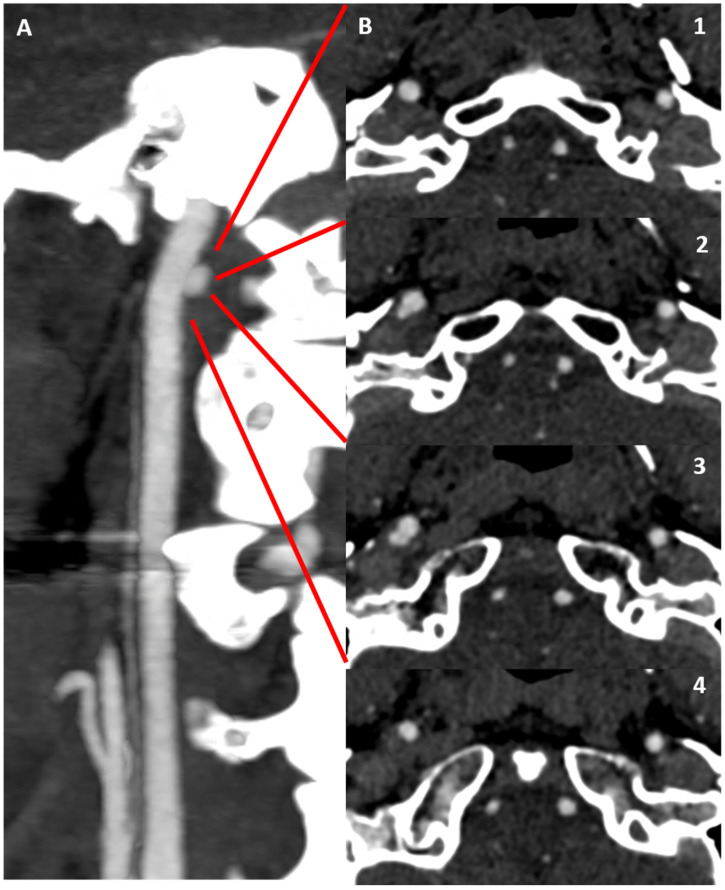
CTA focused on the right ICA. Panel (A) shows the MIP/MPR reconstructed oblique plane along the course of the right distal extracranial ICA with a huge ulceration of the surface of the known atheroma. In panel (B) are proposed the corresponding axial source images at various levels along the atheroma.
